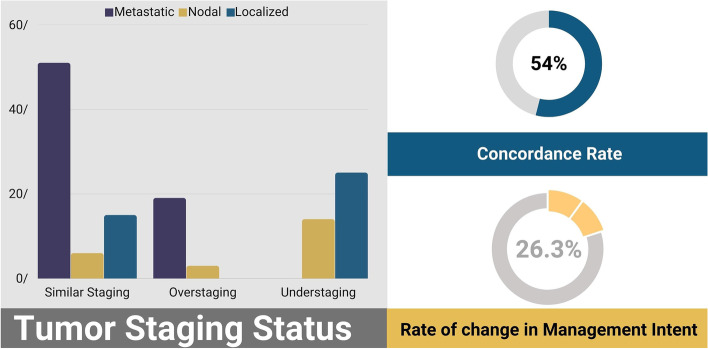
Overall concordance rate and patterns of change in Staging and management achieved through PET/CT compared against whole-body CT
